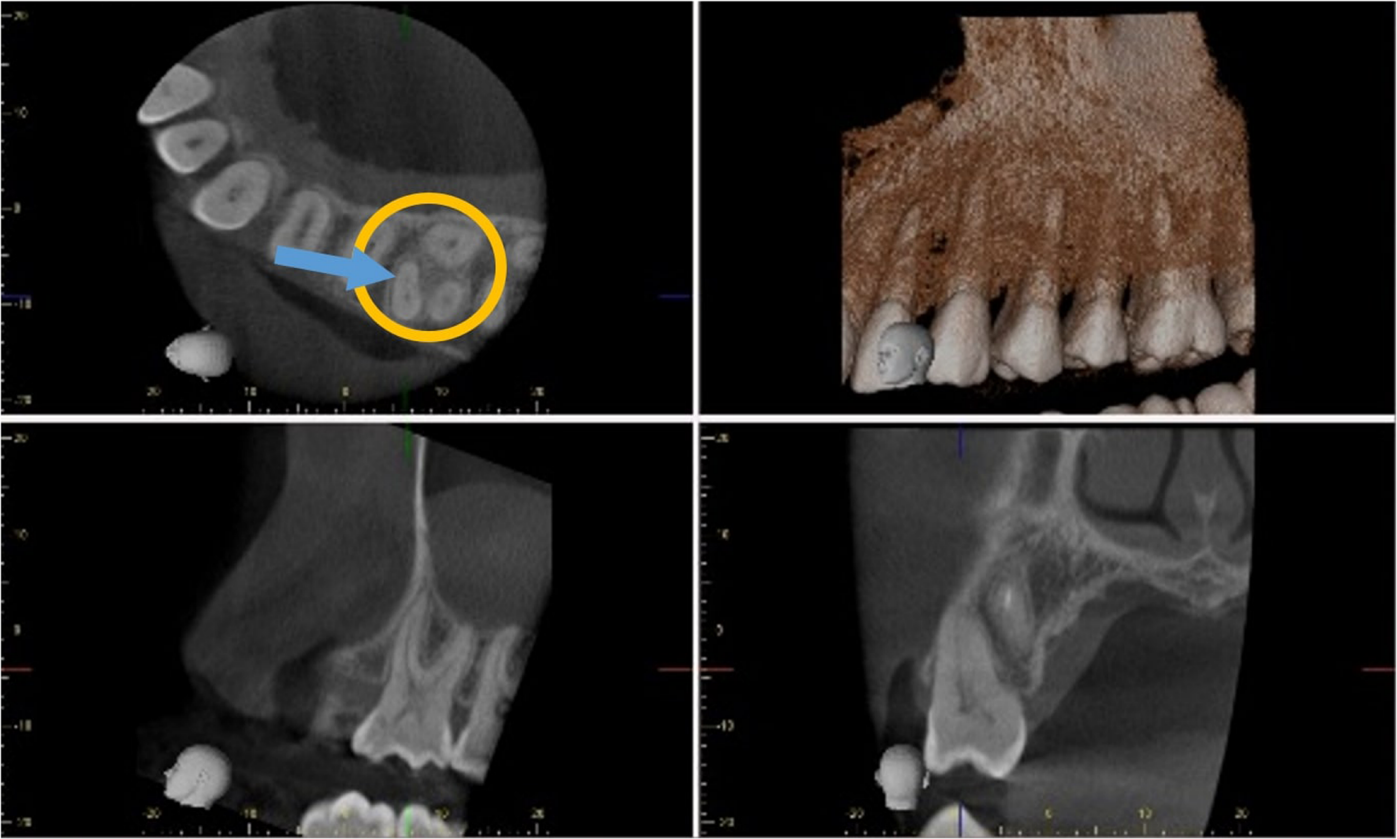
CBCT scan of teeth in the upper left maxillary region. A distinct MB-2 canal (blue arrow) on an axial cross section of an upper first molar (yellow circle).
